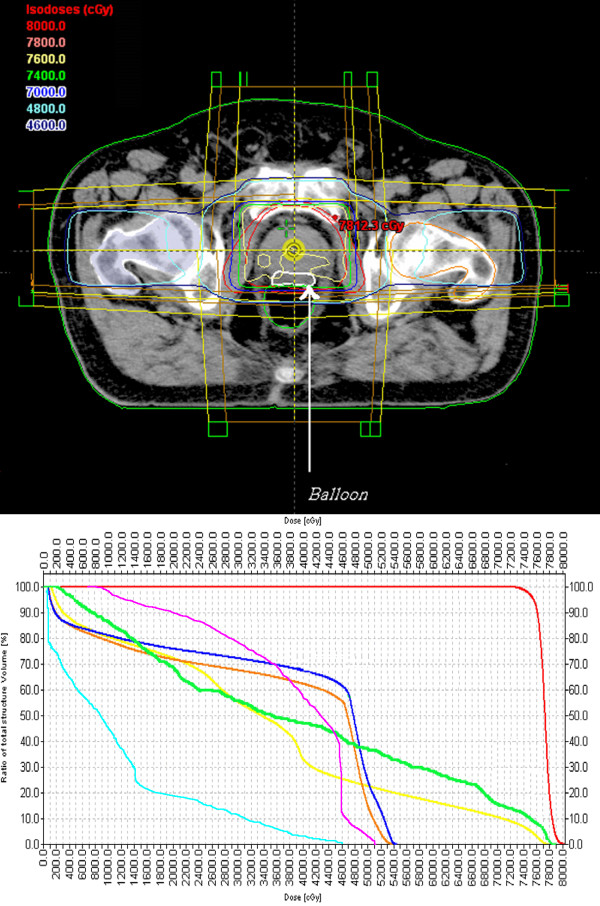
CT plane with isodose distribution. The Prospace is outlined in white (arrow). 3a: with balloon; 3b: without balloon.
